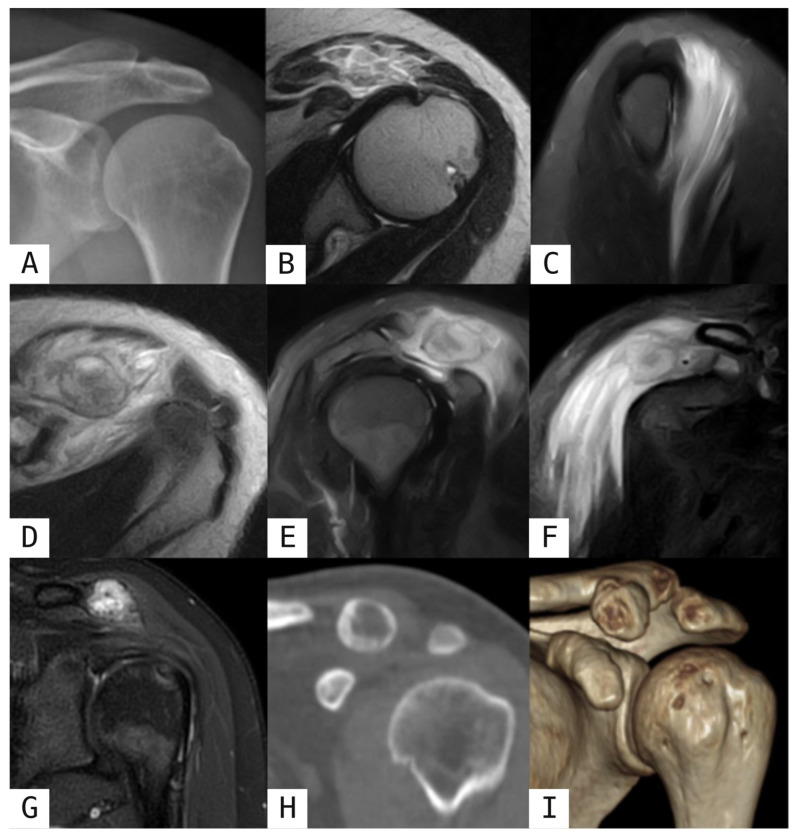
Myositis ossificans in the left deltoid muscles. A 28-year-old woman presented with two weeks of non-traumatic left shoulder pain. X-rays were unremarkable (A). MRI axial T2-weighted (B) and sagittal STIR (C) images showed diffuse oedema and enlargement of the anterior deltoid with the checkerboard pattern on the axial plane and the striated pattern on the sagittal plane. After 5 weeks, follow-up MRI axial T2-weighted (D), sagittal STIR (E) and coronal STIR sequences (F) revealed a 2.5 cm oval lesion in the proximal anterior deltoid, adjacent to the distal clavicle and coracoid process. The lesion had a hypointense peripheral rim and was surrounded by muscle oedema. Six months post-onset, MRI coronal PD fat-sat (FS) sequence (G) showed muscle oedema resolution and multiplanar CT coronal reconstruction (H) and 3D rendering (I) revealed the lesion’s peripheral ossification.
